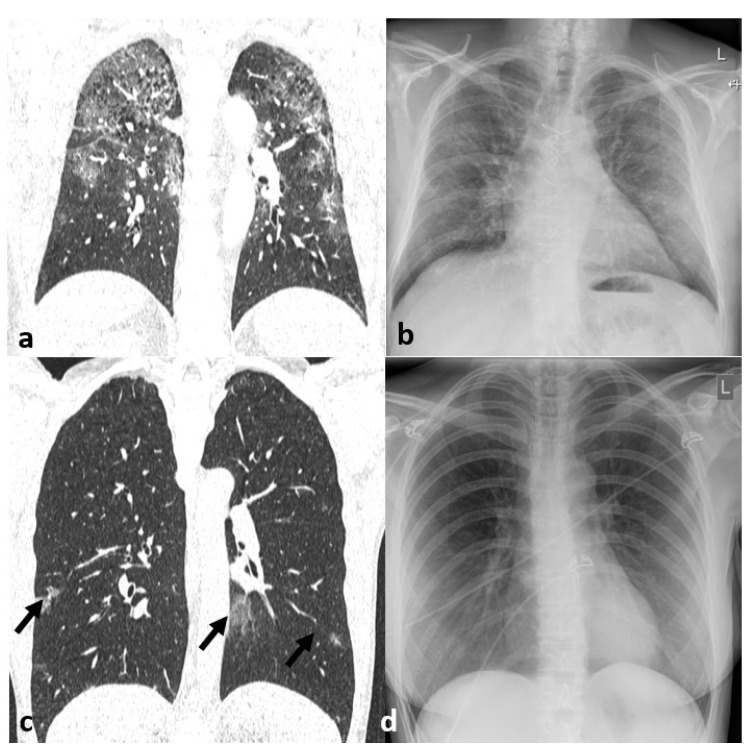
(a,b) 66-year-old male COVID-19 patient presenting with ground-glass opacification (GGO) predominantly in the upper lobes on (a) chest computed tomography comprising between 25 and 50% of the lung parenchyma. (b) Conventional radiography which was obtained 4 h before CT showed only subtle opacification in the left upper lobe and probably pneumonia affecting less than 25% of the lung parenchyma was given. (c,d) 55-year-old female COVID-19 patient presenting with subpleural ground-glass opacification (GGO) on (c) chest computed tomography (arrows) affecting less than 25% of the lung parenchyma. (d) Conventional radiography that was obtained 2 h before CT showed no suspicious changes and was rated as normal.
